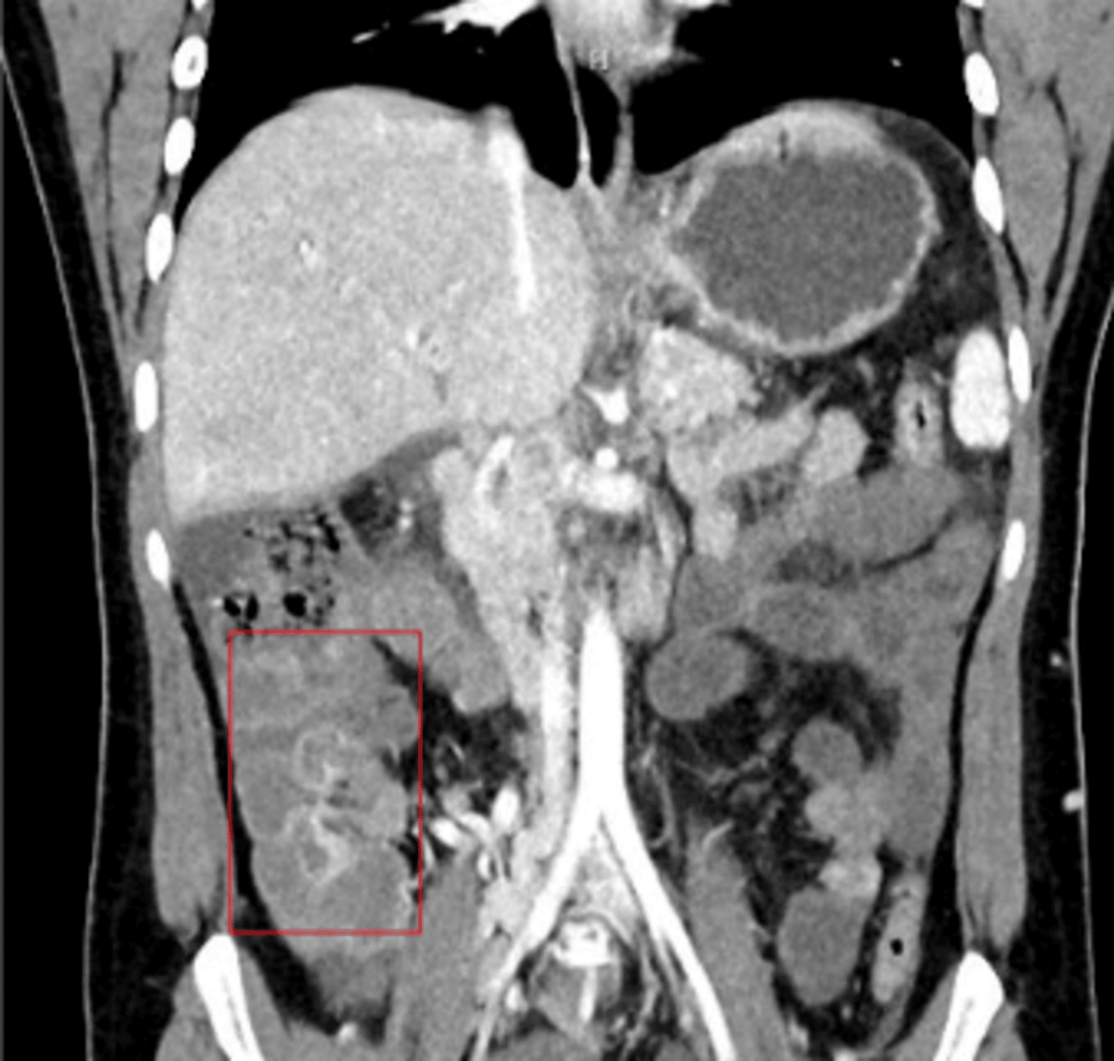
CT abdomen/pelvis showing increased small bowel wall thickening and hyperenhancement of loops of bowel (red box)
